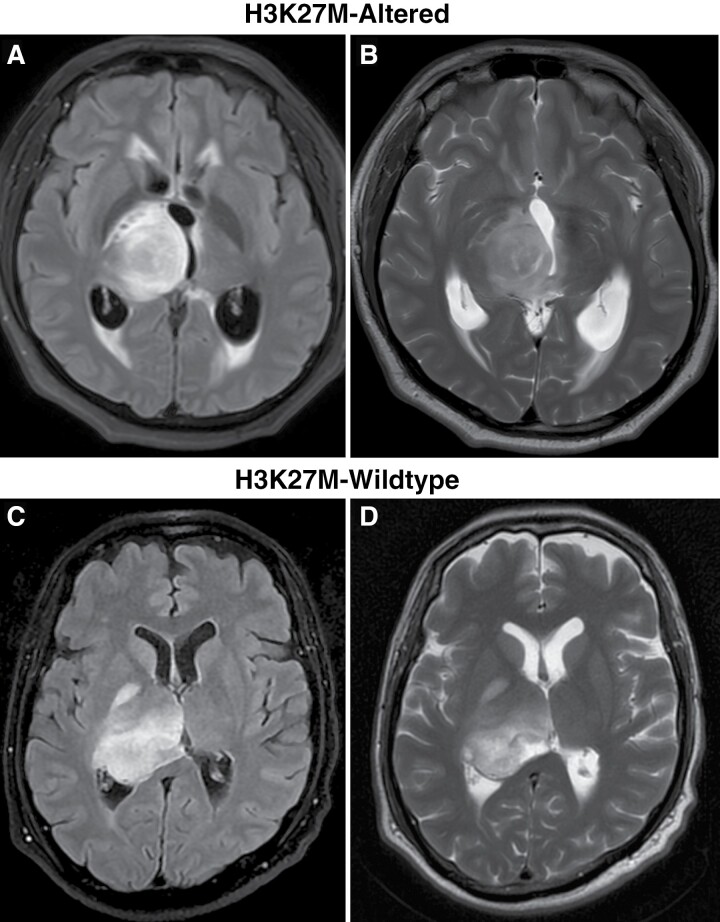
Images for H3K27M-altered and H3K27M-wild-type subjects. (A) T2W-FLAIR image for H3K27M-altered subject. (B) T2W image for H3K27M-altered subject. (C): T2W-FLAIR image for H3K27M-wild-type subject. (D) T2W image for H3K27M-wild-type subject.
